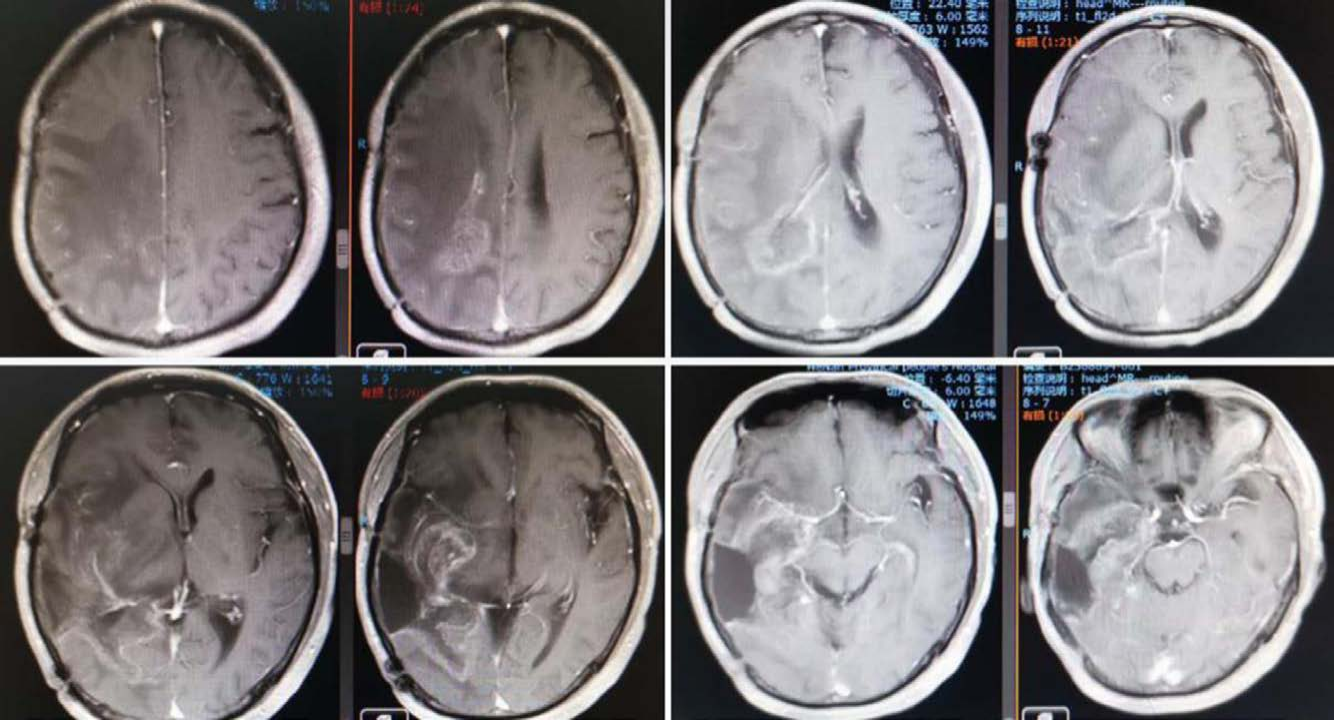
On December 14, 2016, the magnetic resonance imaging of the head showed postoperative changes of glioma, abnormal enhancement of the right temporo-occipital lobe, and possible recurrence.
